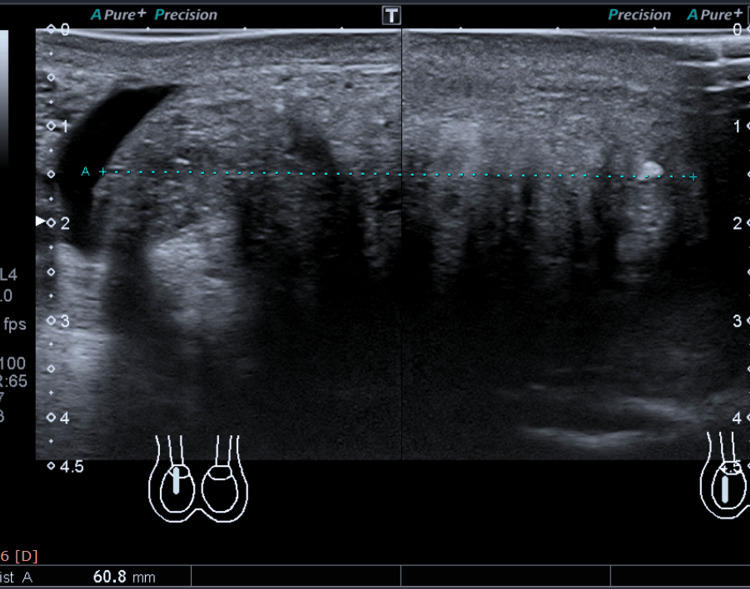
Pre-treatment scrotal ultrasound.
